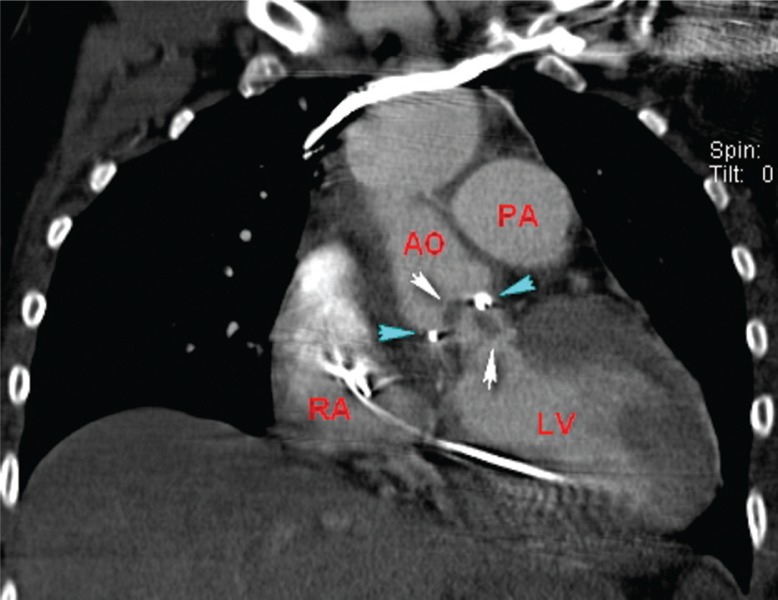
Coronal view from a contrast-enhanced thoracic computed tomography scan demonstrates the extensive vegetations (white arrows) on the aortic valve prosthesis (blue arrows). The right atrium (RA), left ventricle (LV), ascending aorta (AO), and main pulmonary artery (PA) are noted.
